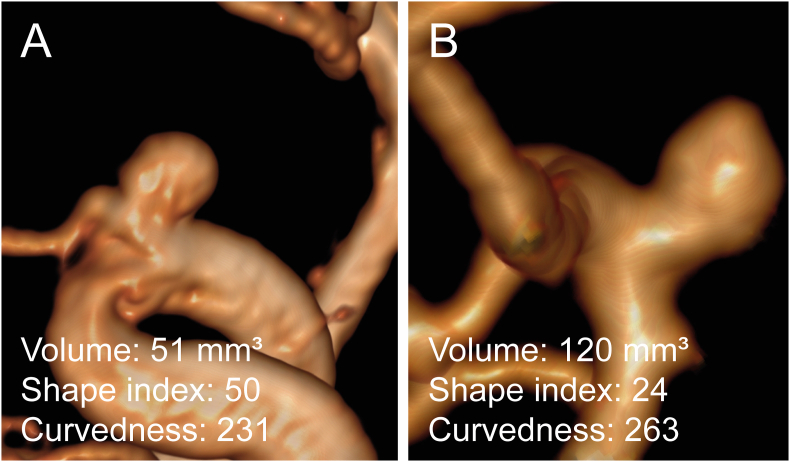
Example unruptured intracranial aneurysms in a woman and a man. Multiplanar reconstructions of unruptured intracranial aneurysms. a 34-year old woman with 4-mm aneurysm of the right internal carotid artery on MR angiography. b 52-year old man with 7-mm aneurysm of the left internal carotid artery on CT angiography. Aneurysms in women generally had lower volumes, higher shape index, and lower curvedness values compared to men. Sphericity, shape index, and curvedness were multiplied by 100.
